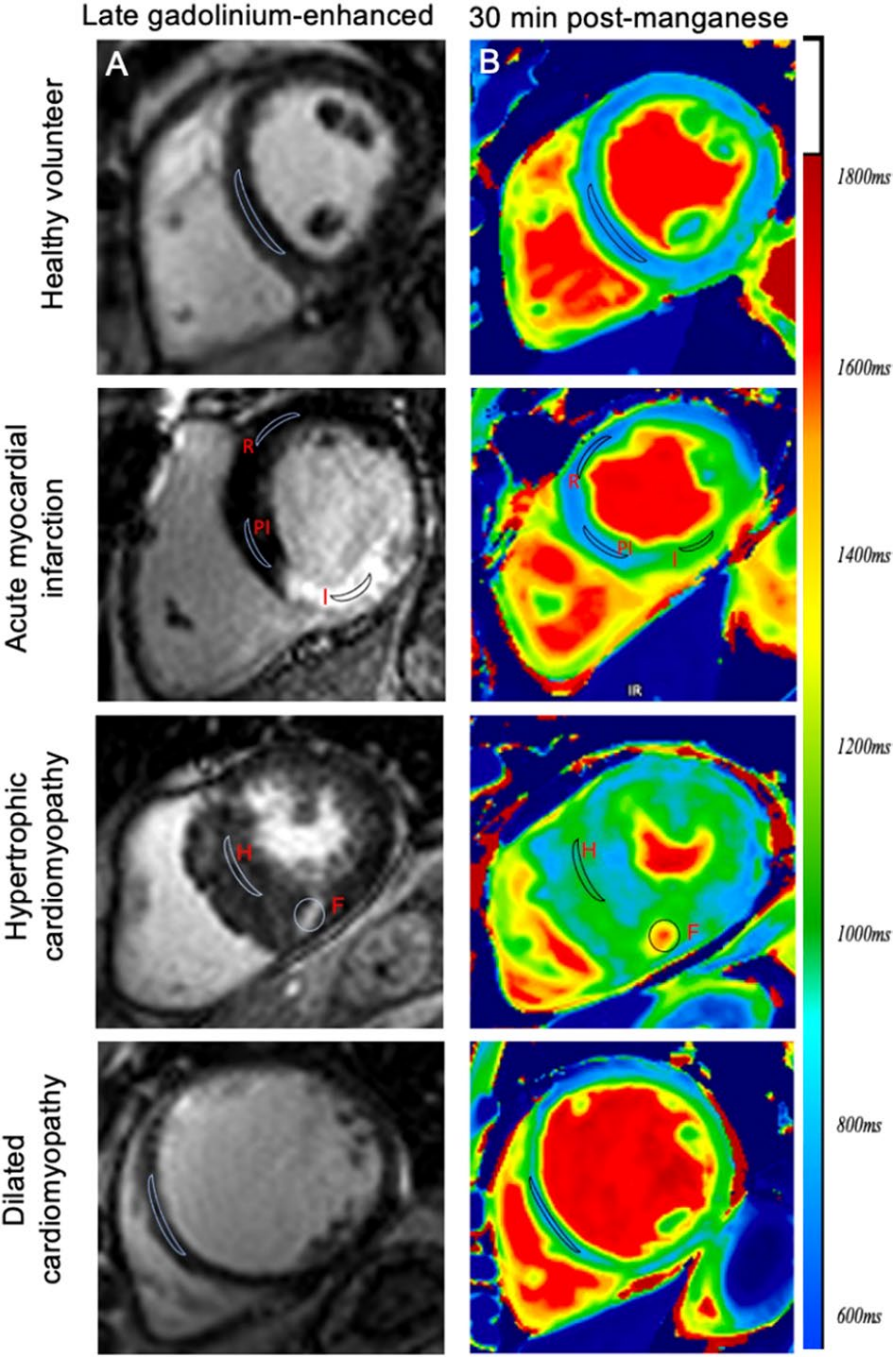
Regions of interest in managanese-enhanced magnetic resonance imaging. Late-gadolinium enhanced (A) and 30 min post-manganese T1 maps (B) in healthy volunteers, patients with acute myocardial infarction, hypertrophic and dilated cardiomyopathy. Demonstrating regions of interest in healthy volunteers (septum) and patients with acute myocardial infarction (infarct, I, peri-infarct, PI, and remote, R), hypertrophic cardiomyopathy (hypertrophied, non-fibrotic, H and fibrotic, F) and dilated cardiomyopathy (septum).
